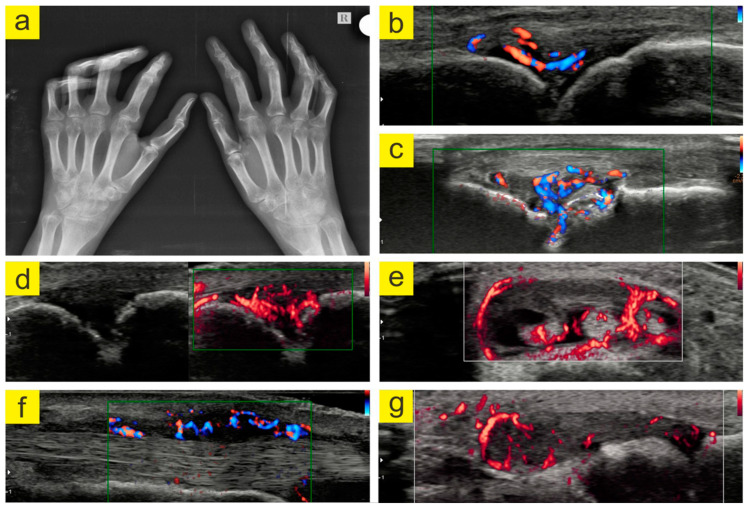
(a). Bilateral AP hand radiography. Radiological imaging identified multiple marginal erosions; narrowing of the proximal and distal interphalangeal, metacarpophalangeal, carpo-metacarpal, and carpal bone joints in both hands; osteoporosis in the band; subluxation proximal interphalangeal II, III, IV, and V of the left hand; proximal interphalangeal IV and V of the right hand, and metacarpophalangeal IV and V of the right hand. (b). Hyper vascular synovial pannus (directional power Doppler). (c). Synovial pannus and bone erosion (directional power Doppler). (d). Hyper vascular synovial pannus (microvascular flow). (e). Tenosynovitis extensor tendons of the fingers (microvascular flow). (f). Synovial pannus radial extensor of the carpus and ulnar extensor of the carpus. (g). Synovial pannus at the MTF I joint level (microvascular flow). (Images archive of the first author).
